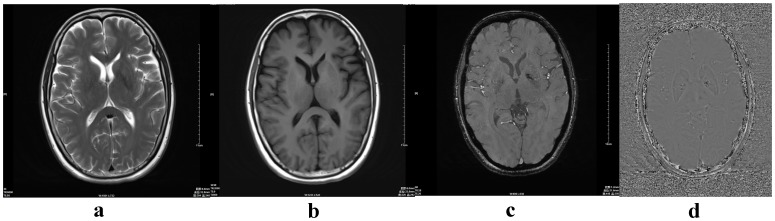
A 58-year-old normal male. (a) axial T2-weighted image; (b) axial T1-weighted image; (c) magnitude image; (d) phase image.
